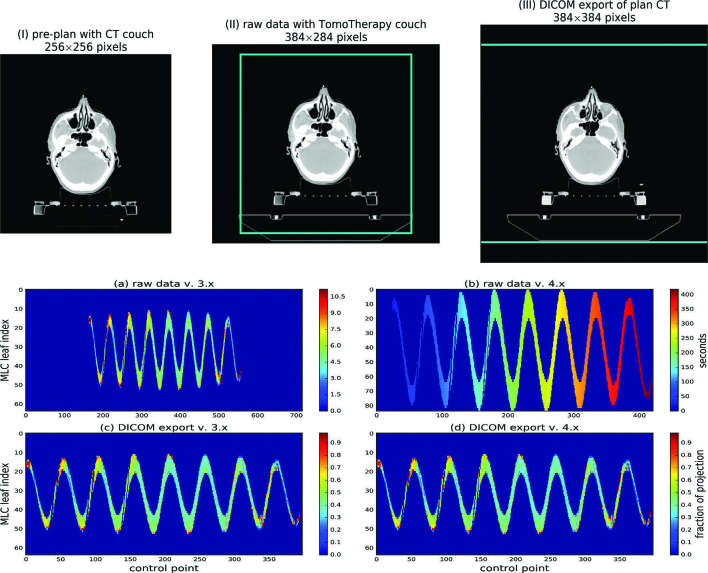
Transformations of binary data for the DICOM export. (I –III) Insertion of the treatment machine couch image into the plan CT. (I) 256 × 256 CT image with CT scanner couch; (II) treatment couch is inserted, the image is enhanced to 384 × 284 pixels to incorporate a larger couch image with preservation of the treatment isocentre; (III) for DICOM export, the image is padded to 384 × 384 pixels. Cyan lines in (II) and (III) mark the image area inherited from the previous processing step. (a –d) Sinogram export in vv 3.xx (a, c) and 4.xx (b, d). (a, b): raw data in vendor storage. (b, d) RTPLAN Tomo Projection Sinogram Data tag (300 D, 10A7). Raw data for v.4.x (b) show leaf opening and closing times for the MLC leaves open during the treatment (MLCs 11 to 52); the sinogram height is number of open leaves × 2. The entries for leaves 1 –10 and 53 –64, that are closed for the entire treatment duration, are not recorded. The left hand-side of (b) depicts 10 s beam warm-up time.
